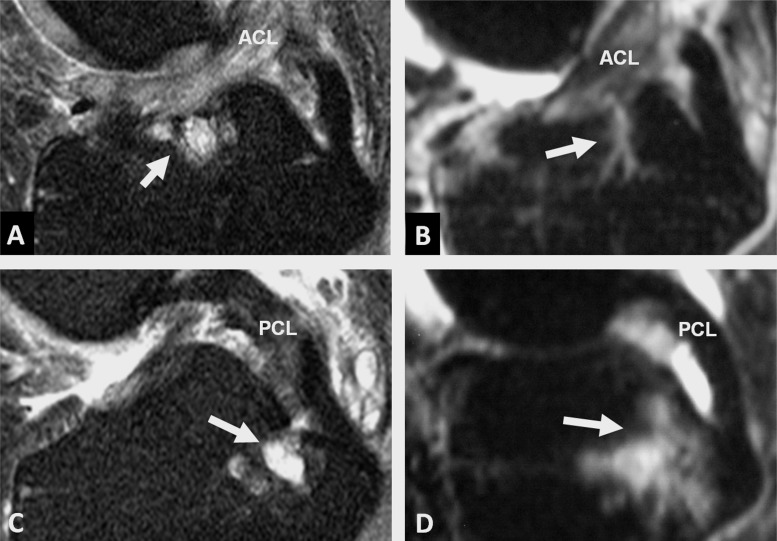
Sagittal fluid-sensitive MRI images showing oedematous features (arrows) adjacent to the vascular channels in patients with osteoarthritis (OA) and inflammatory arthritis. Bone marrow lesions adjacent to the anterior cruciate ligament (ACL) vascular channel in A, patient with early OA and B, patient with undifferentiated arthritis. Bone marrow lesions and intraosseous cysts adjacent to the posterior cruciate ligament (PCL) vascular channel in C, patient with early OA and D, patient with psoriatic arthritis.
